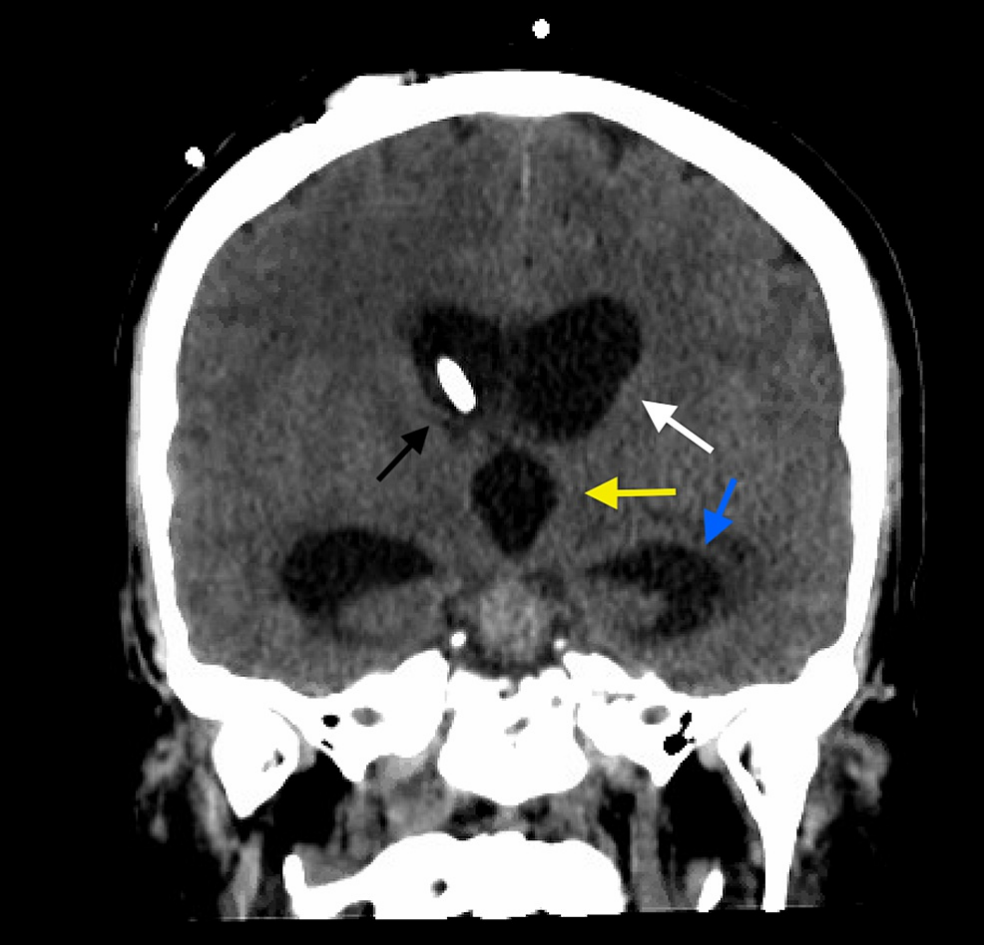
Coronal non-contrasted CT head demonstrating ventriculomegaly of the third lateral ventricle (white arrow), third ventricle (yellow arrow), and temporal horns (blue arrow) despite functioning EVD terminating in right foramen of Monro (black arrow). CT, computed tomography; EVD, external ventricular drain.
